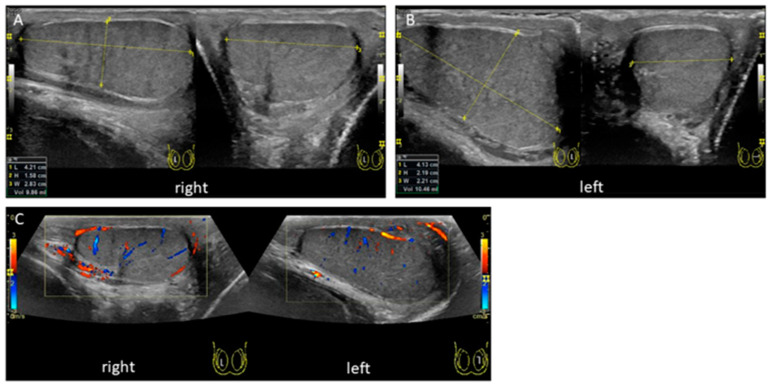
Ultrasonography 8 weeks after detorsion. An ultrasound was performed 8 weeks after the operation. The right testicle recovered its parenchymal structure and size (A). Left testicle is shown in (B). Regular central perfusion was observed equally on both sides (C).
